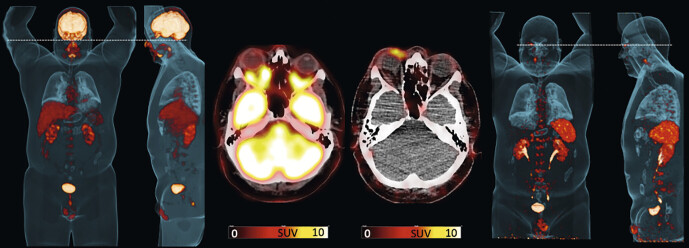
Maximum intensity projections of PET/CTs using [18F]FDG (leftmost panels) and [68Ga]Ga-PentixaFor (rightmost panels) in a subject with orbital marginal zone lymphoma. The middle panels show PET/CT images of axial sections of lymphoma manifestation using [18F]FDG (bottom) and [68Ga]Ga-PentixaFor (top). On [18F]FDG, MZL manifestation is not visible due to the physiological high uptake of the PET probe in brain tissue, whereas the [68Ga]Ga-PentixaFor-based images enables detection of the manifestation in the periorbital area. Data from same patient but different images as in Buck et al, J Nucl Med 2023 Jul;64(7):1009–1016
